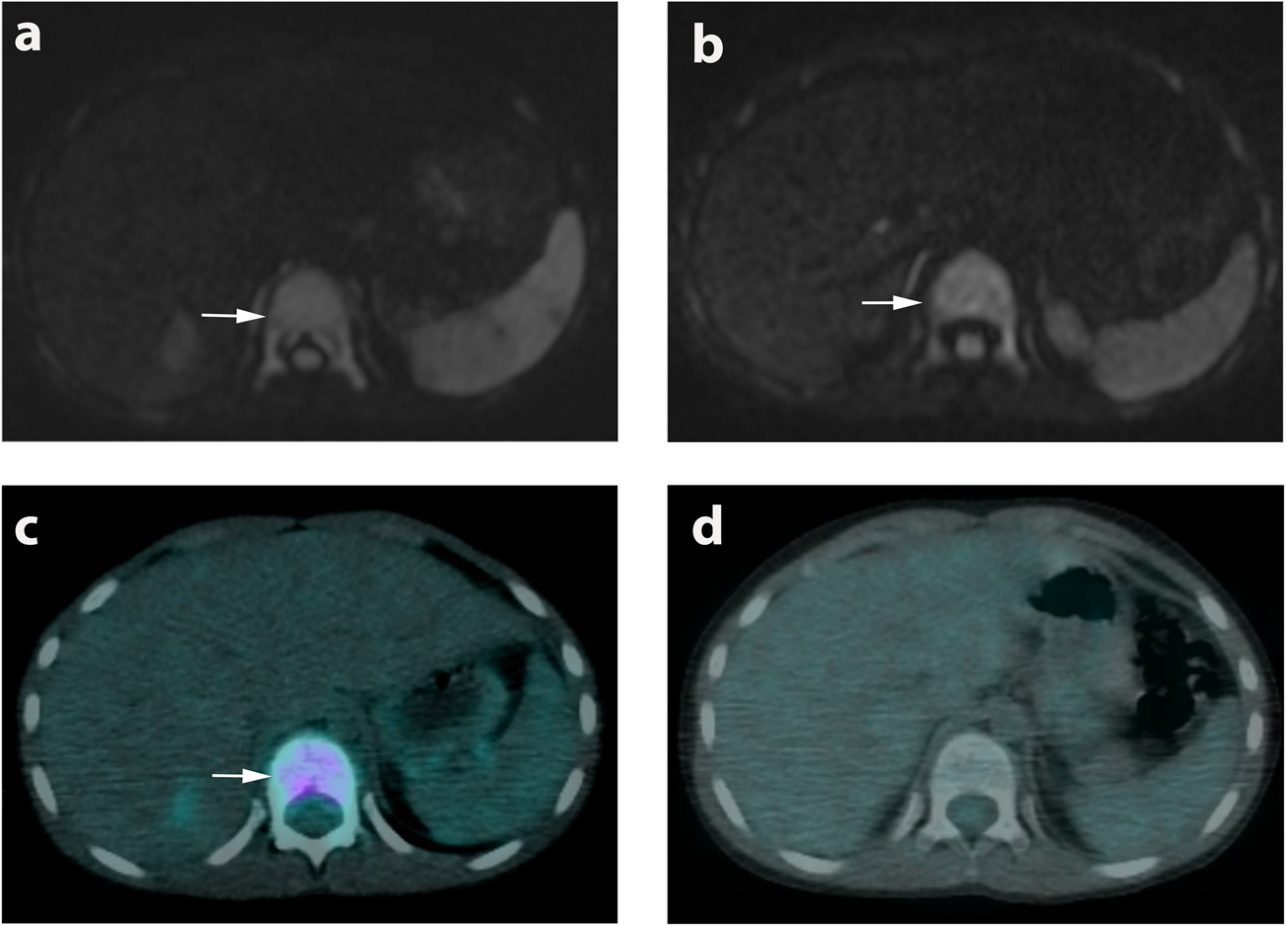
Example of discrepant interim treatment response classification. WB-MRI and ^18^F-FDG-PET-CT of an 8-year-old male subject with Ann Arbor stage 4 disease. Baseline WB-MRI (a) and ^18^F-FDG-PET-CT (c) showing involvement of entire T11 vertebrae (arrows). Interim WB-MRI (b) showing no signal intensity changes (arrow) whilst interim ^18^F-FDG-PET-CT (d) demonstrated complete response. Patient remained in remission following chemotherapy
